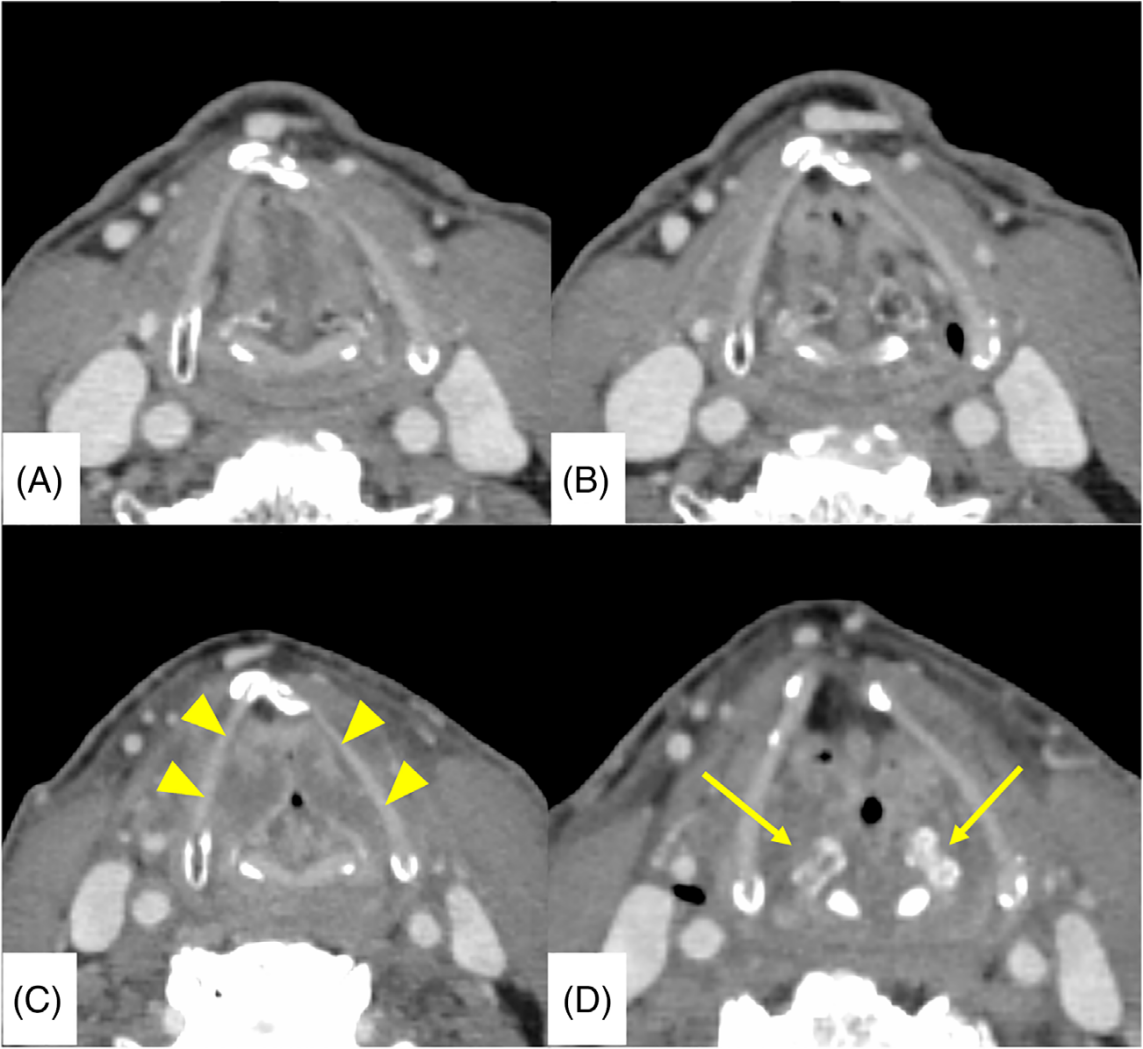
Comparison of computed tomography (CT) axial images obtained before treatment (A, B) and at the time of total laryngeal paralysis (C, D). Post‐treatment CT reveals severe edema in the bilateral vocal cord (arrow head). Bilateral arytenoid cartilage shows atrophy and sclerosis (arrow). There was no recurrence of the primary cancer. These findings indicated late radiation toxicity
